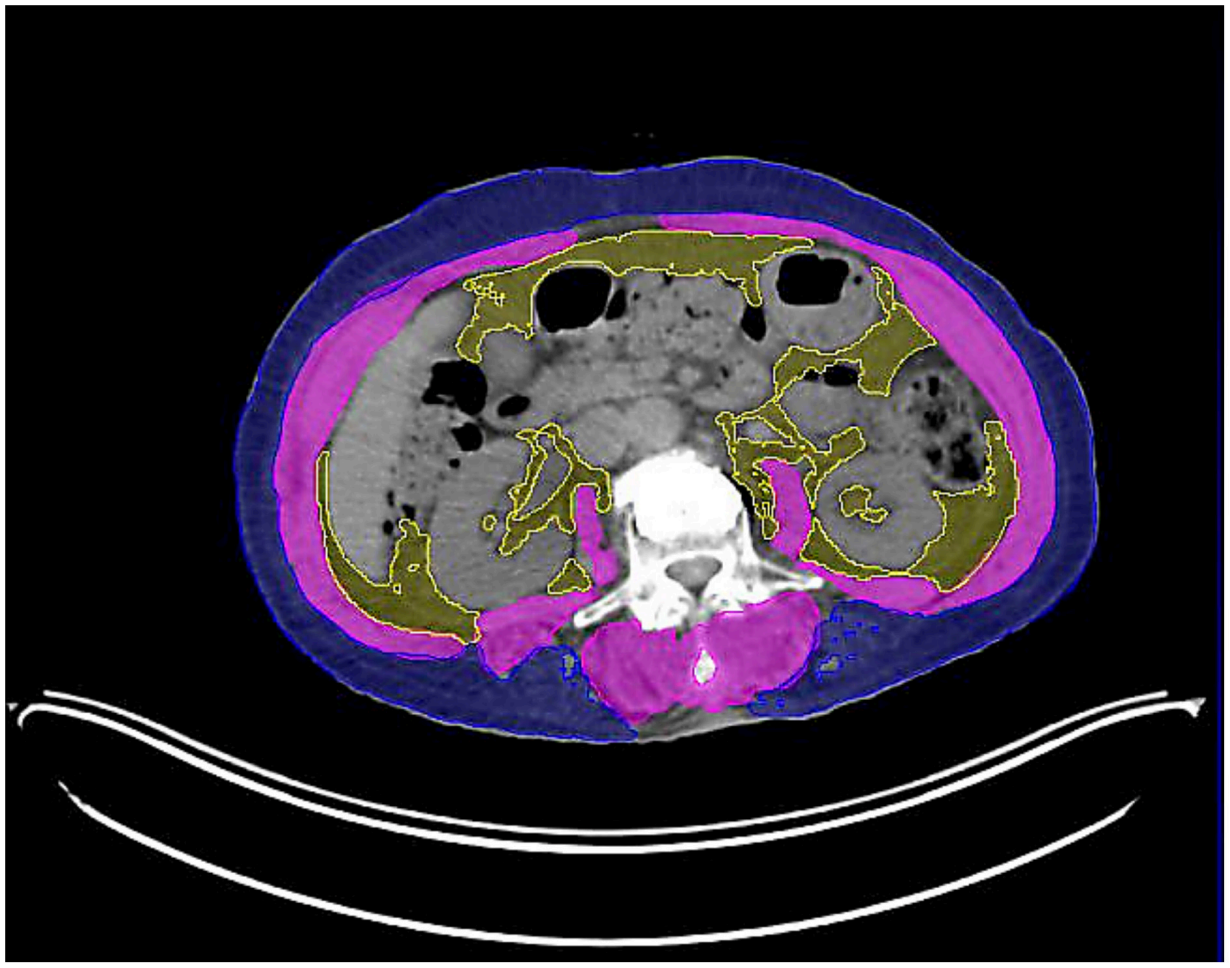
Axial computed tomography image of the third lumbar vertebra used for the assessment of skeletal muscle area (highlighted in pink).
